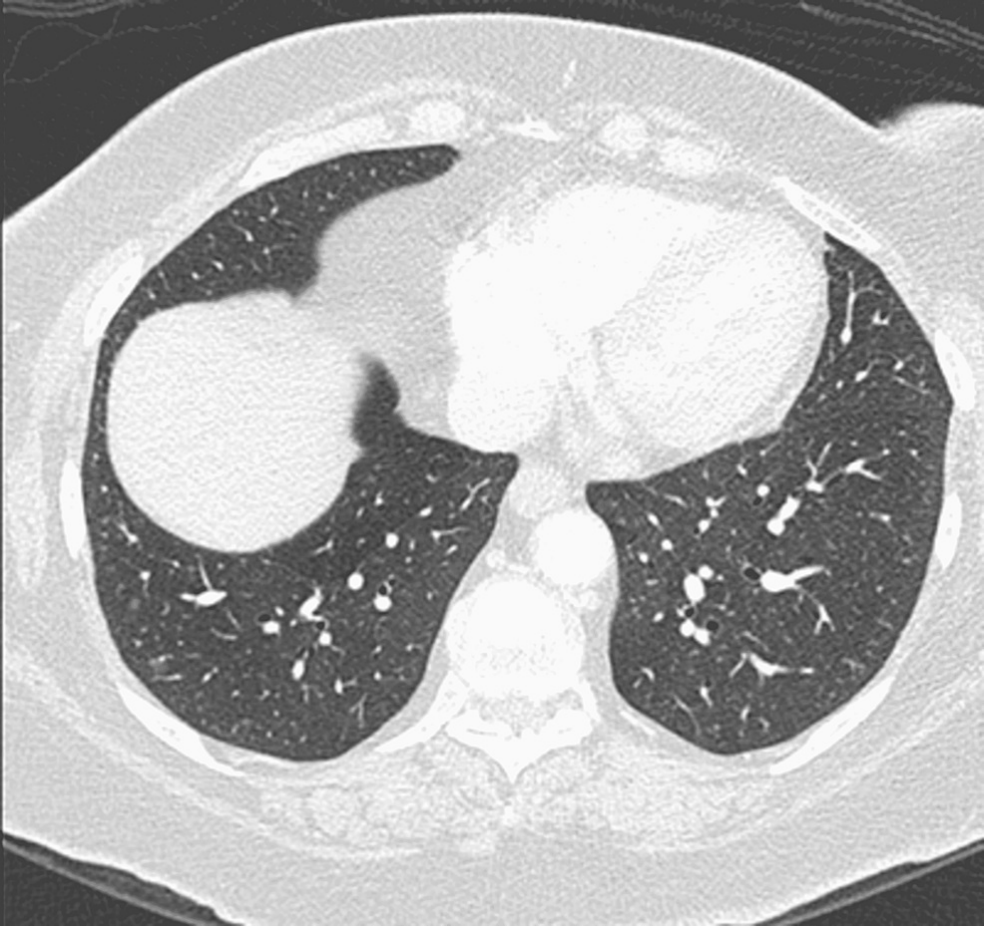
Systemic involvement of Rosai-Dorfman disease of the breast Computed tomography with contrast (a part of positron emission tomography-computed tomography scan (PET-CT)) exhibited small, low-density, nodular, and ground-glass opacities at both lung bases, thought to be representative of Rosai-Dorfman disease.
